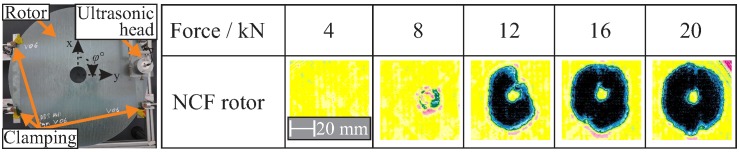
Overview of the ultrasonic results after out-of-plane compression loads with different force magnitudes for NCF rotors, where the formation of delaminations is observed by 8 kN.
